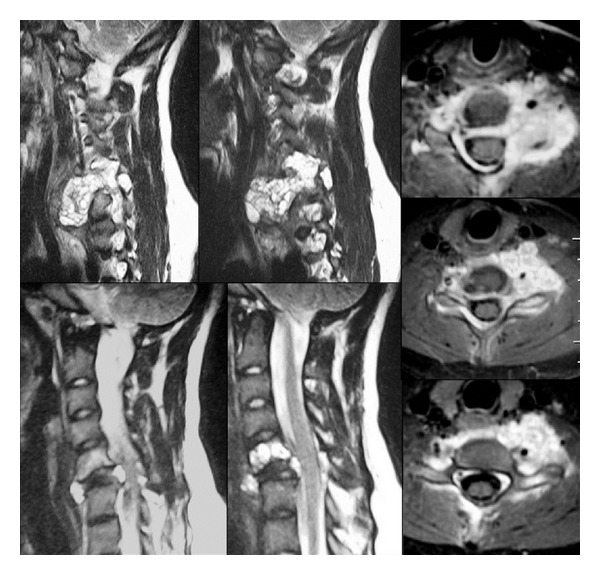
MRI scans of the cervical spine, showing a “soap-bubble” lesion of C5 and C6 with anterolateral paraspinal extension, which encaged both vertebral arteries and spinal cord.
